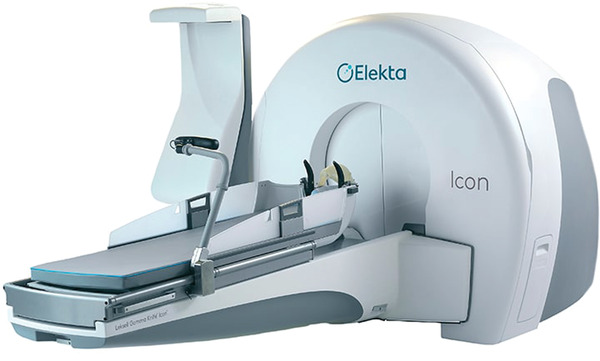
The Gamma Knife Icon with its base delivery system, mask immobilization, high‐definition motion management (HDMM), and cone‐beam computed tomography (CBCT) (Image courtesy of Elekta) © 2018 Elekta AB
